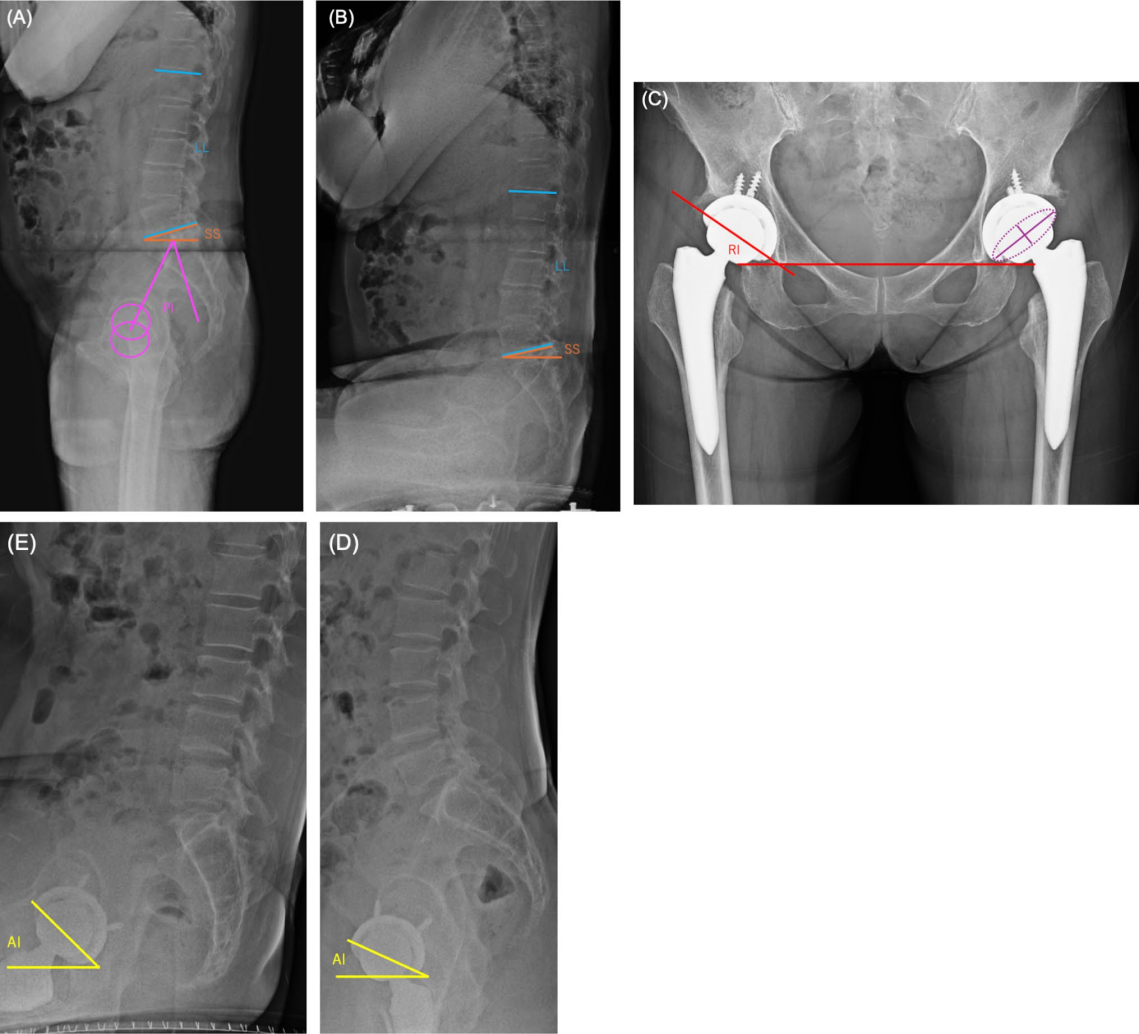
Lateral view showing the spinopelvic parameters. The pelvic incidence (PI) is the angle between the line perpendicular to the sacral endplate and the line connecting the middle of the sacral endplate to the midpoint of the bicoxofemoral axis (pink). The sacral slope (SS) is the angle between the horizontal plane and the sacral endplate (orange). The lumbar lordosis (LL) is the angle between the superior endplate of L1 and sacral endplate (blue). (A and B), Radiographic inclination (RI) is the angle between a vertical line and a line through the long axis of its ellipse (red) on the anteroposterior plane. Radiographic anteversion (RA) is the arcsin of the short axis of the ellipse drawn perpendicular to the long axis of the component or maximum diameter (purple). (C) Anteinclination (AI) is the angle between a vertical line and a line through the long axis of its ellipse (yellow) on the sagittal plane. (D and E)
